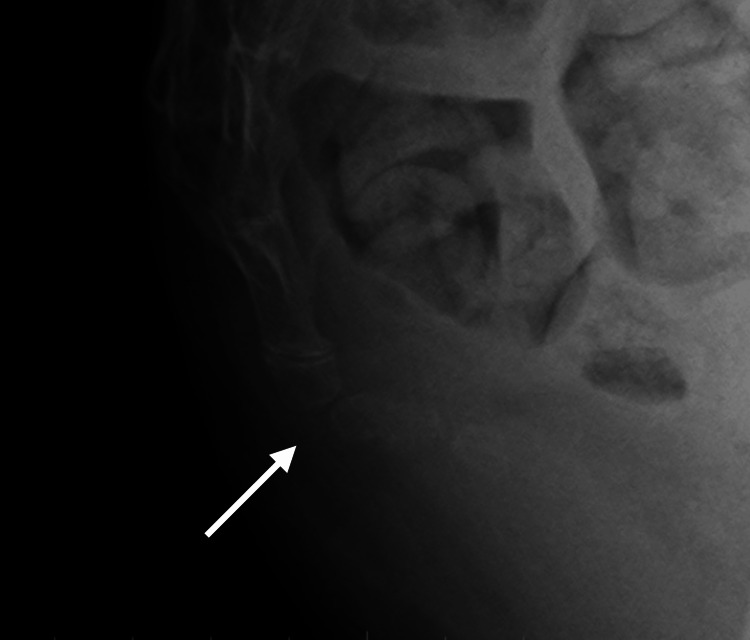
Coccy X-ray imaging The white arrow indicates suspected sacroiliac joint dislocation, although there is no tenderness.
